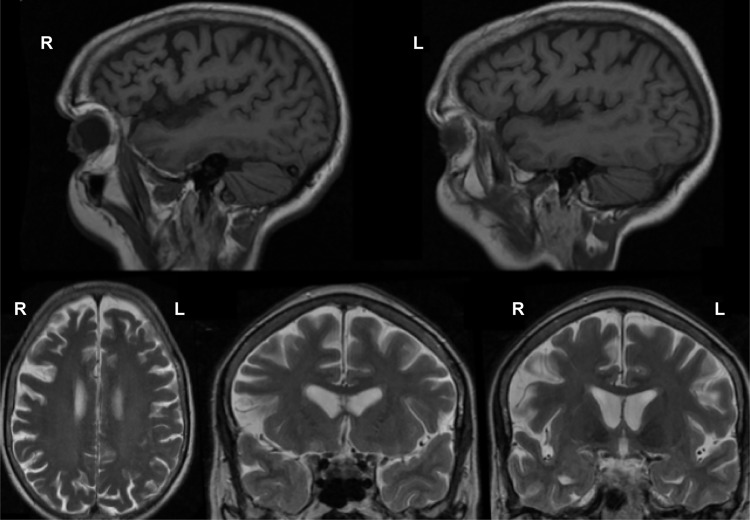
Magnetic resonance imaging scans demonstrating frontal lobe atrophy, with the right side more markedly affected. (*Top*) T1-weighted sagittal views. (*Bottom*) T2-weighted axial (*left*) and coronal (*middle* and *right*) views.
